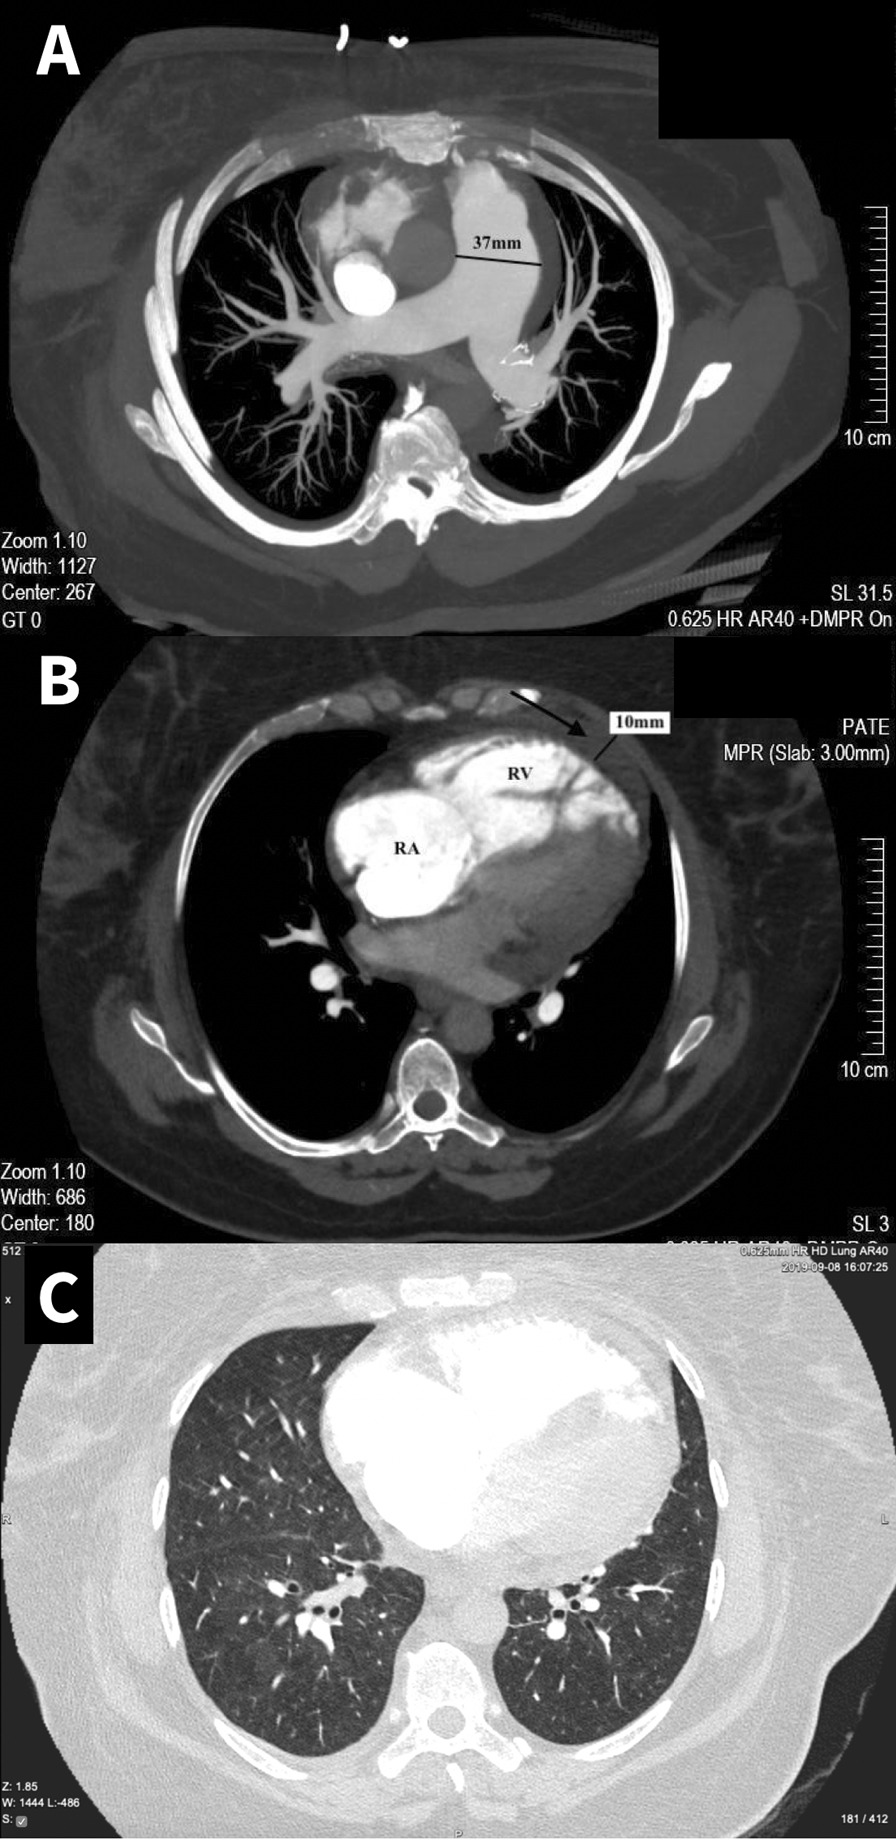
Reconstruction of computerized tomography pulmonary angiography (CTPA). Axial CT angiography scans: A the dilatation of the pulmonary artery trunk and both main pulmonary arteries with no filling defects within pulmonary vasculature. B The dilatation of right ventricle (RV), atrium (RA) and pericardial effusion (black arrow). C multiple perivascular areas of hyperattenuation in keeping with PH. Note the increased segmental artery-to-bronchus ratio up to 2,5:1
